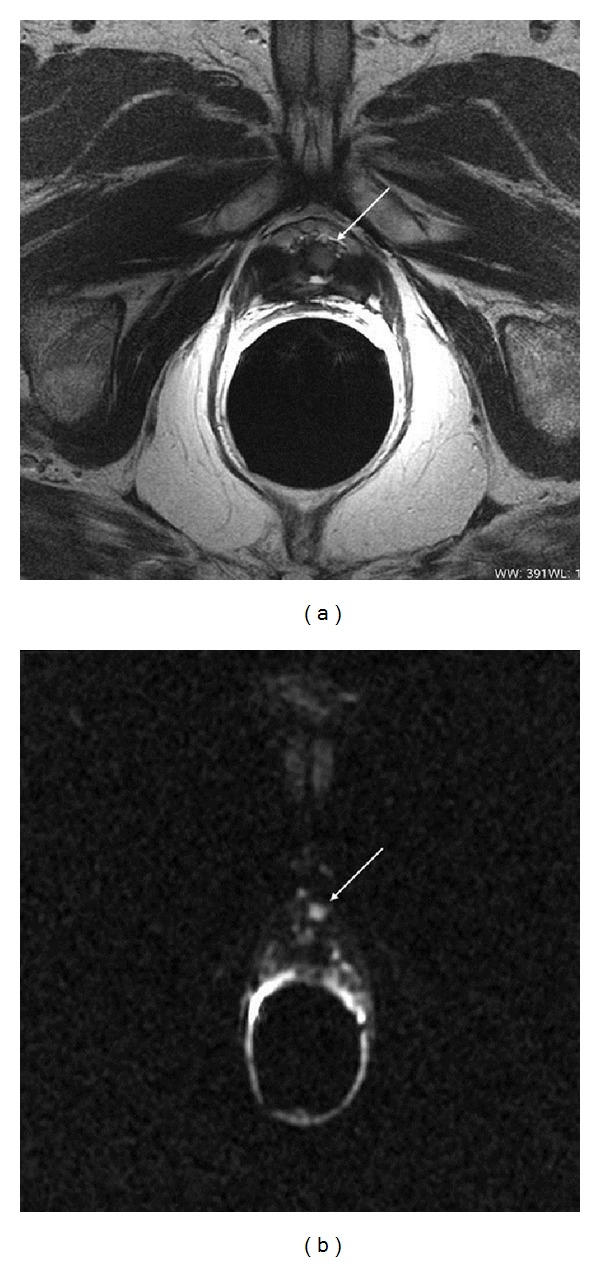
Relapse after radical prostatectomy. Arrows show a nodular intermediate signal intensity in the T2-weighted image (a), characterized by hyperintensity in DWI (score DWI = 1: (b)).
